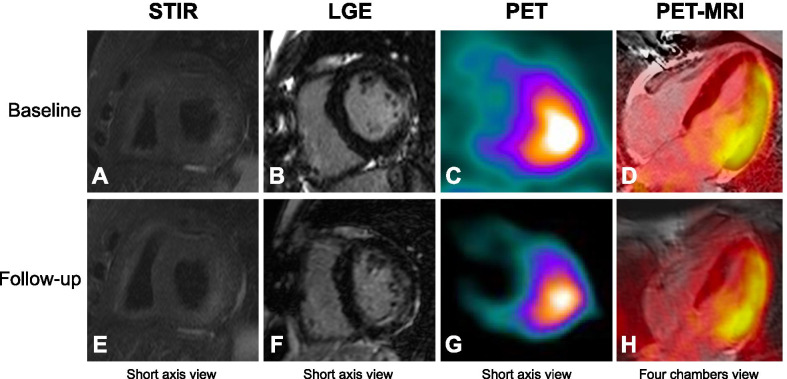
50-year-old female (patient #12) with focal hyperintensity on STIR image (A) and focal LGE in the basal segments of the lateral wall of the LV (B), increased ^18^F-FDG uptake in the corresponding myocardial region (C) and relative fused images (D) at baseline study. No significant changes of PET-MRI findings at follow-up study (E–H)
